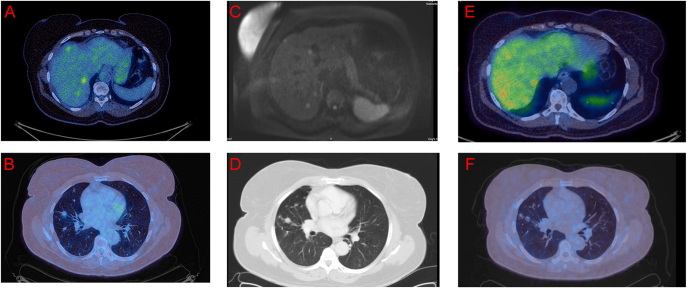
(A and B) ^18^F-FDG PET images from case 1, demonstrating low-grade avidity in the liver metastases (A) and dominant nodules (B). (C) MRI liver diffusion-weighted imaging identifies a number of small liver metastases. (D) CT thorax demonstrating a DIPNECH with dominant nodules. (E) ^68^Ga-DOTATATE PET imaging demonstrating avidity in some of the liver metastases. (F) ^68^Ga-DOTATATE PET imaging demonstrating low-grade avidity in the lung.
